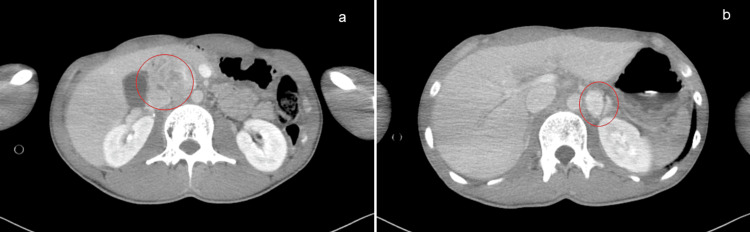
Axial CT with intravenous contrast Axial CT with intravenous contrast demonstrate a 50 mm tumour anterior to the inferior vena cava (a) and a 20 mm enhancing tumour in the left adrenal gland (b).
